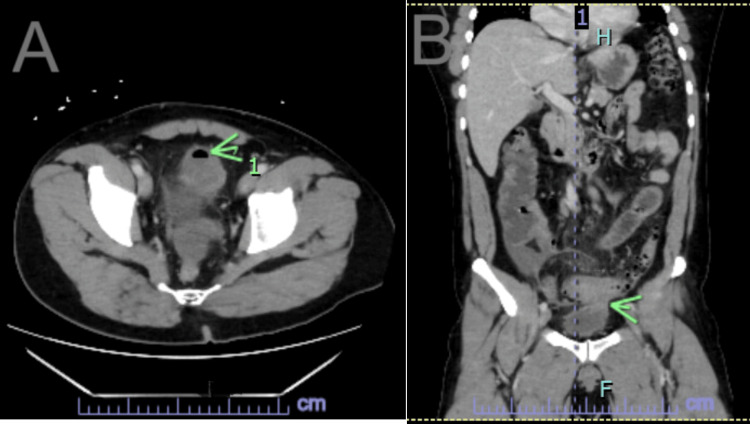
CT of colovesical fistula (A) Transverse; (B) Coronal
